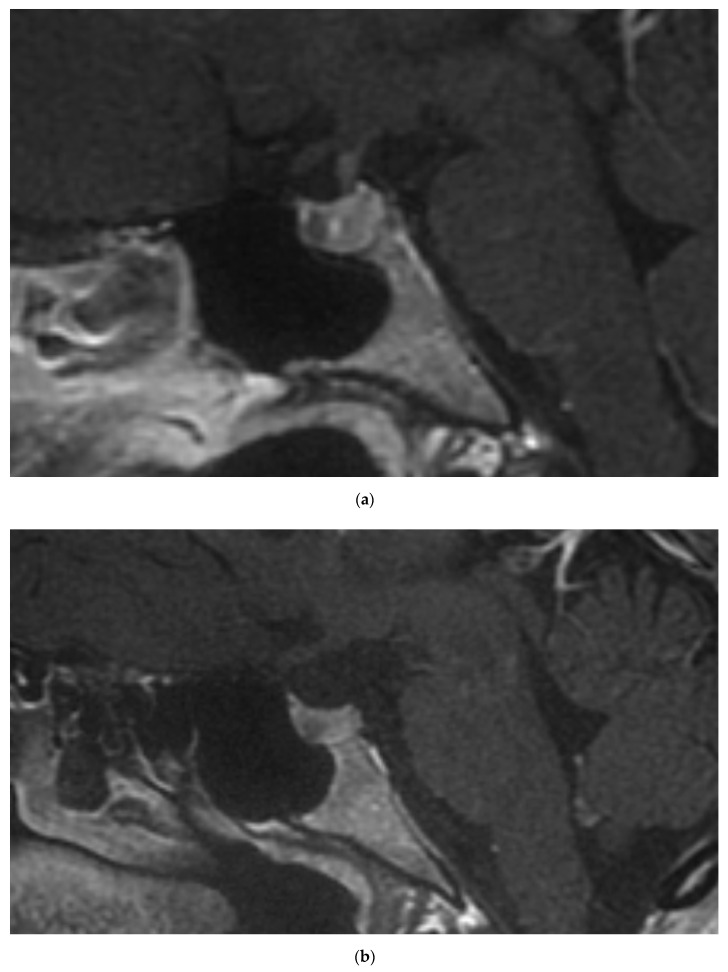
Contrast-enhanced magnetic resonance imaging revealed pituitary microadenomas in patient 2 (a) and patient 3 (b).
